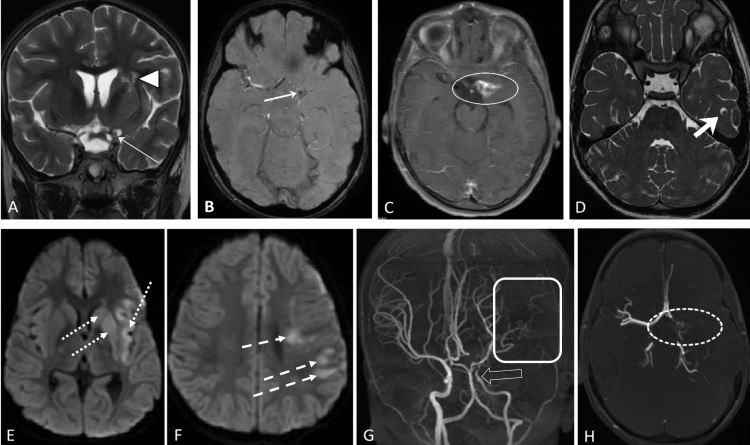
MRI and MRA brain. MRI brain, plain and contrast imaging A) Coronal T2 weighted image showing conglomerated hyperintense cystic lesions with an eccentric hypointense nodule in the left Sylvian fissure (thin white arrow), in the region of the ICA bifurcation, irregular T2 hyper intensity is seen in left putamen, caudate and internal capsule (arrowhead). B) Axial SWAN image shows a tiny hypointensity (thick white arrow) representing calcified mural nodule of the cysticercal cyst. C) Post contrast axial T1 fat-suppressed image showing irregular thick enhancement (solid oval). D) Axial FIESTA-C image showing a cysticercal cyst with eccentric nodule in the left temporal lobe (thick short arrow). E) Axial diffusion weighted image at the level of the basal ganglia showing restricted diffusion lesion, representing infarcts in left MCA territory in the left insula, putamen, and caudate nuclei (dotted white arrows). F) Axial diffusion weighted image at the level of centrum semiovale showing restricted diffusion lesion, representing infarcts in left MCA territory in the left frontal and parietal lobes (dashed arrows). G) MRA, MIP image, showing nonvisualization of left MCA, narrowing of the left supraclinoid ICA (hollow white arrow) and paucity of vessels in the left cerebral hemisphere (white rectangle) compared to the right side. H) Axial thick MIP image showing tiny irregular vessels, possibly representing proliferating collaterals (dashed oval). ICA, internal carotid artery; SWAN, star-weighted angiography; FIESTA, fast imaging employing steady-state acquisition; MCA, middle cerebral artery; MRA, magnetic resonance angiography; MIP, maximum intensity projection; ICA, internal carotid artery
